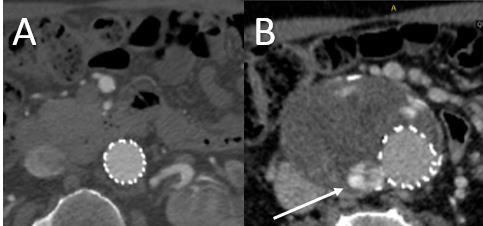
CT after prior conventional EVAR for AAA
